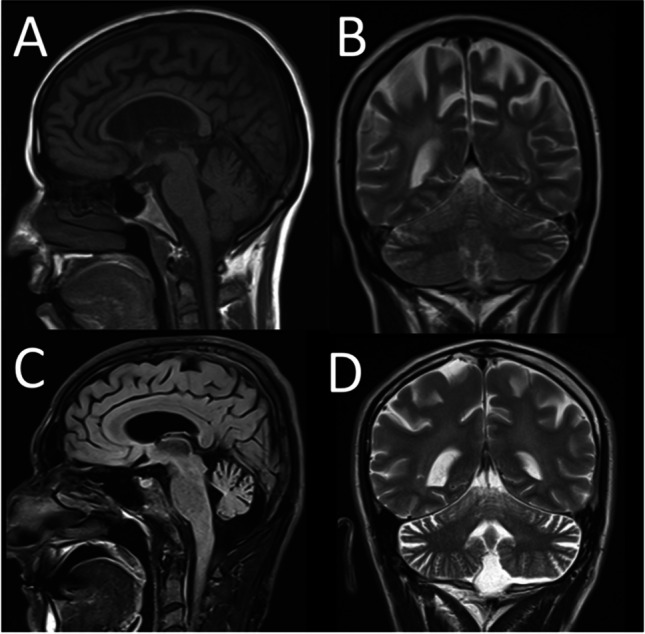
A, B Brain MRI image of patient #25 (sagittal and coronal). C, D Brain MRI image of patient #27 (sagittal and coronal). Both patients have a homozygous variant in the *NPC1* gene and were diagnosed with “Niemann-Pick disease, type C1/D.”
